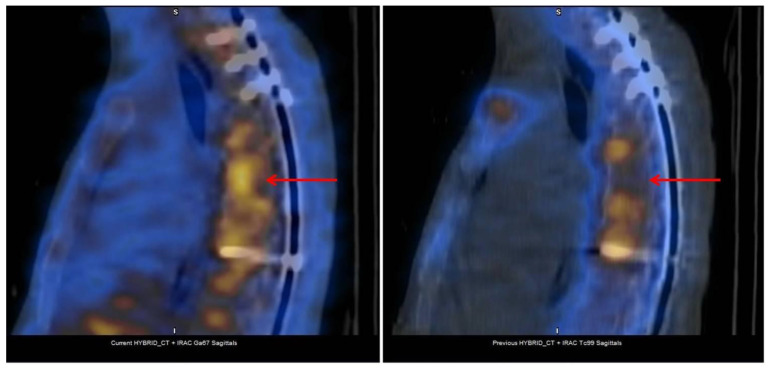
Spondylodiscitis by *Brucella* at T7. A 44-year-old man with dorsal spondylodiscitis by *Brucella*. The patient was previously diagnosed with polyarteritis nodosa and treated with mycophenolate mofetil and glucocorticoids. Combination of scintigraphy with ^99m^Tc-HDP and ^67^Ga with SPECT-CT shows pathological gallium deposits at the level of T7 (red arrows) (axial view) compatible with infectious activity and inflammatory/degenerative bone remodeling changes in the rest of the dorsal vertebral bodies.
